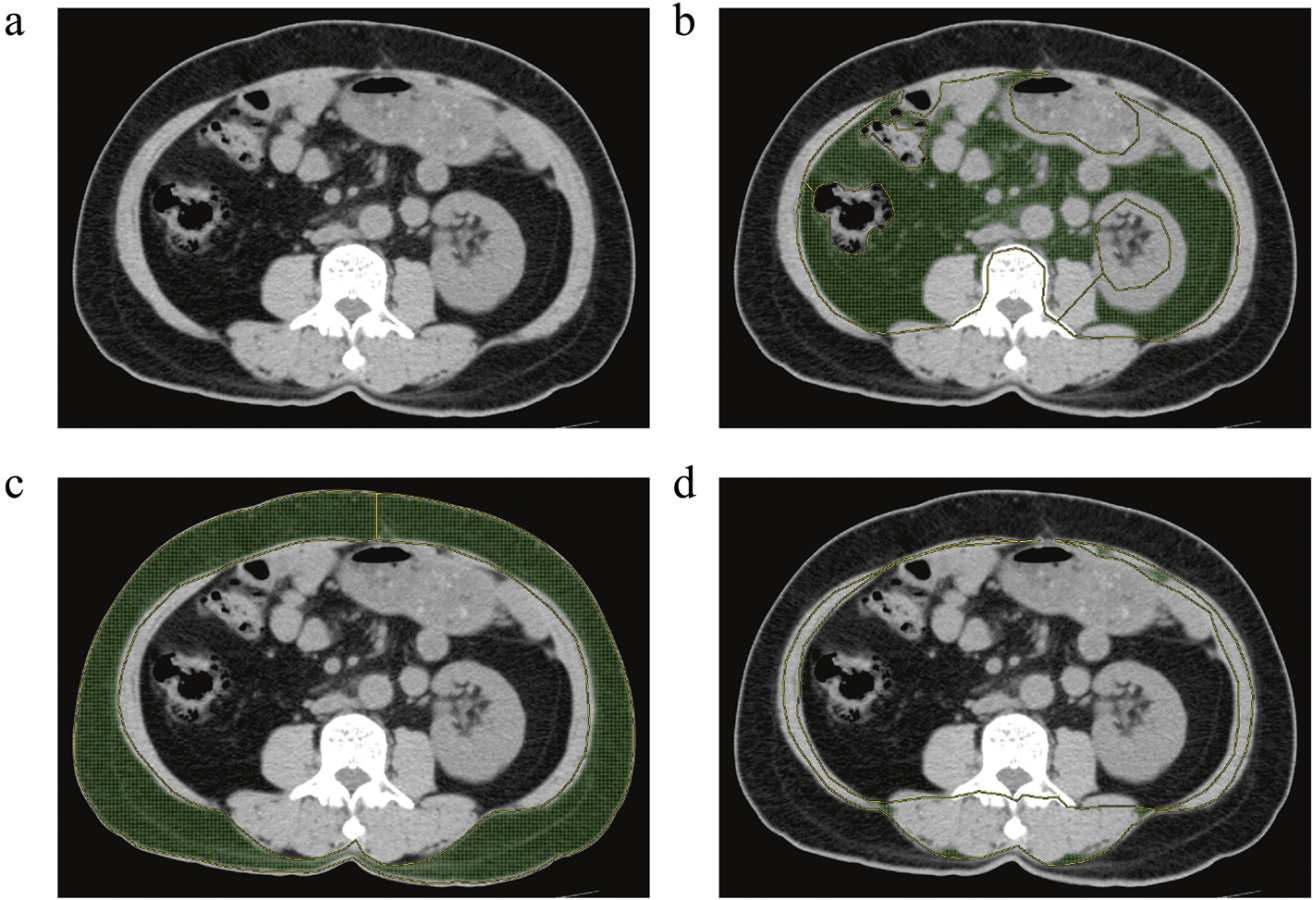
Representative axial CT images with respect to VAT, SAT, and IMAT. a The original CT image of AT and the segmentation of b VAT, c SAT, and d IMAT. CT, computed tomography; AT, adipose tissue; VAT, visceral AT; SAT, subcutaneous AT; IMAT, intermuscular AT
